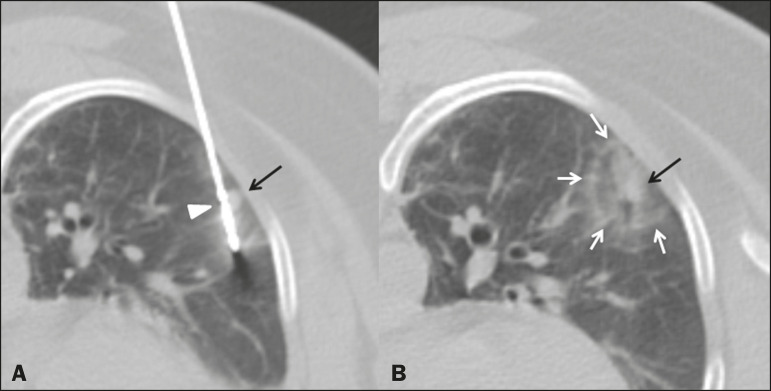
A: Chest computed tomography showing insertion of the microwave antenna (arrowhead) into the secondary pulmonary nodule (arrow). B: Follow-up computed tomography scan showing that the ablated area encompassed the entirety of the treated nodule (black arrow), with satisfactory margins (white arrows).
